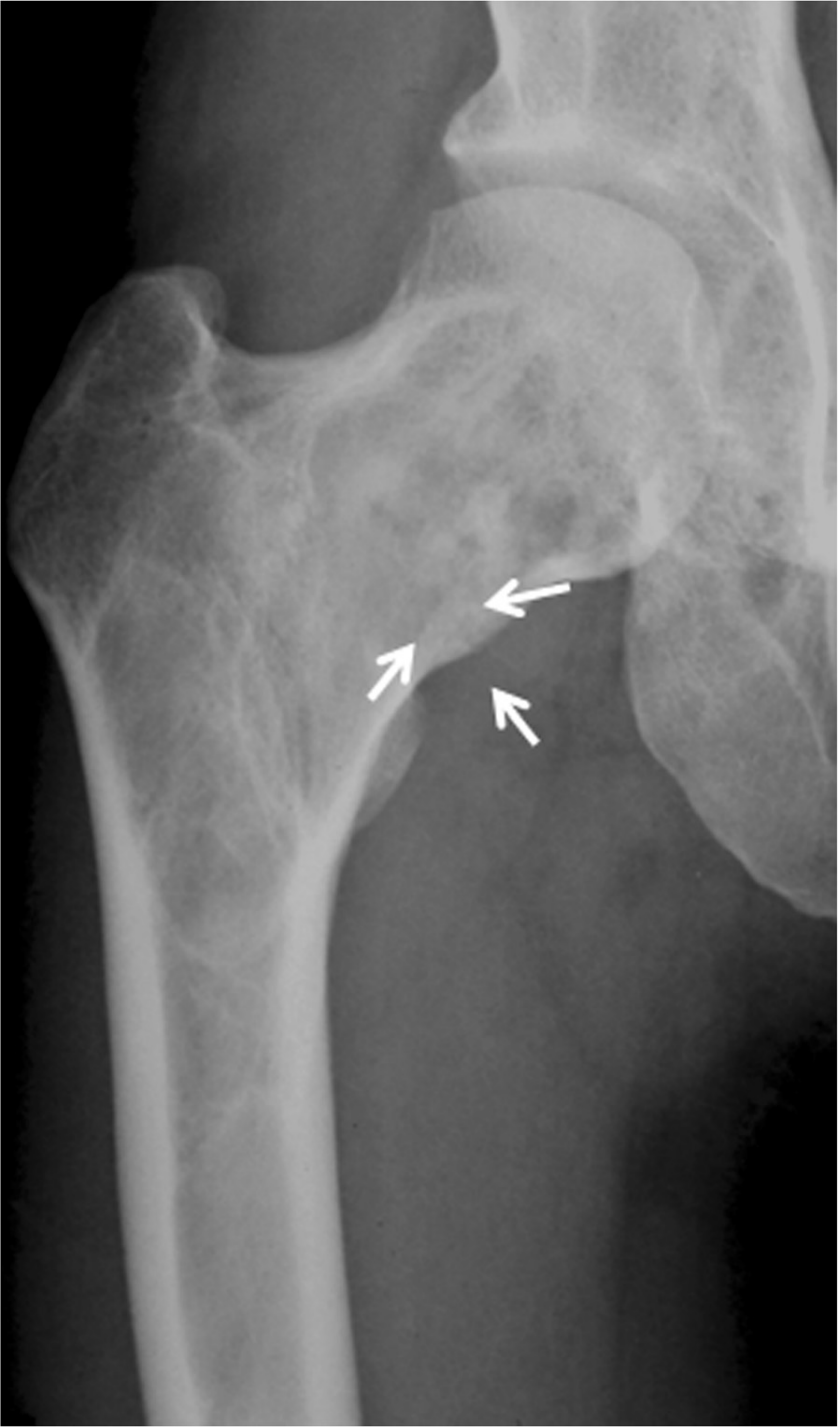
Patient 4. A 32-year-old female presented with repeated bilateral hip pain for 20 years. An A-P X-ray at the time of initial referral indicated fibrous dysplasia of her right femoral neck and “parrot’s beak” sign (*arrows*)
